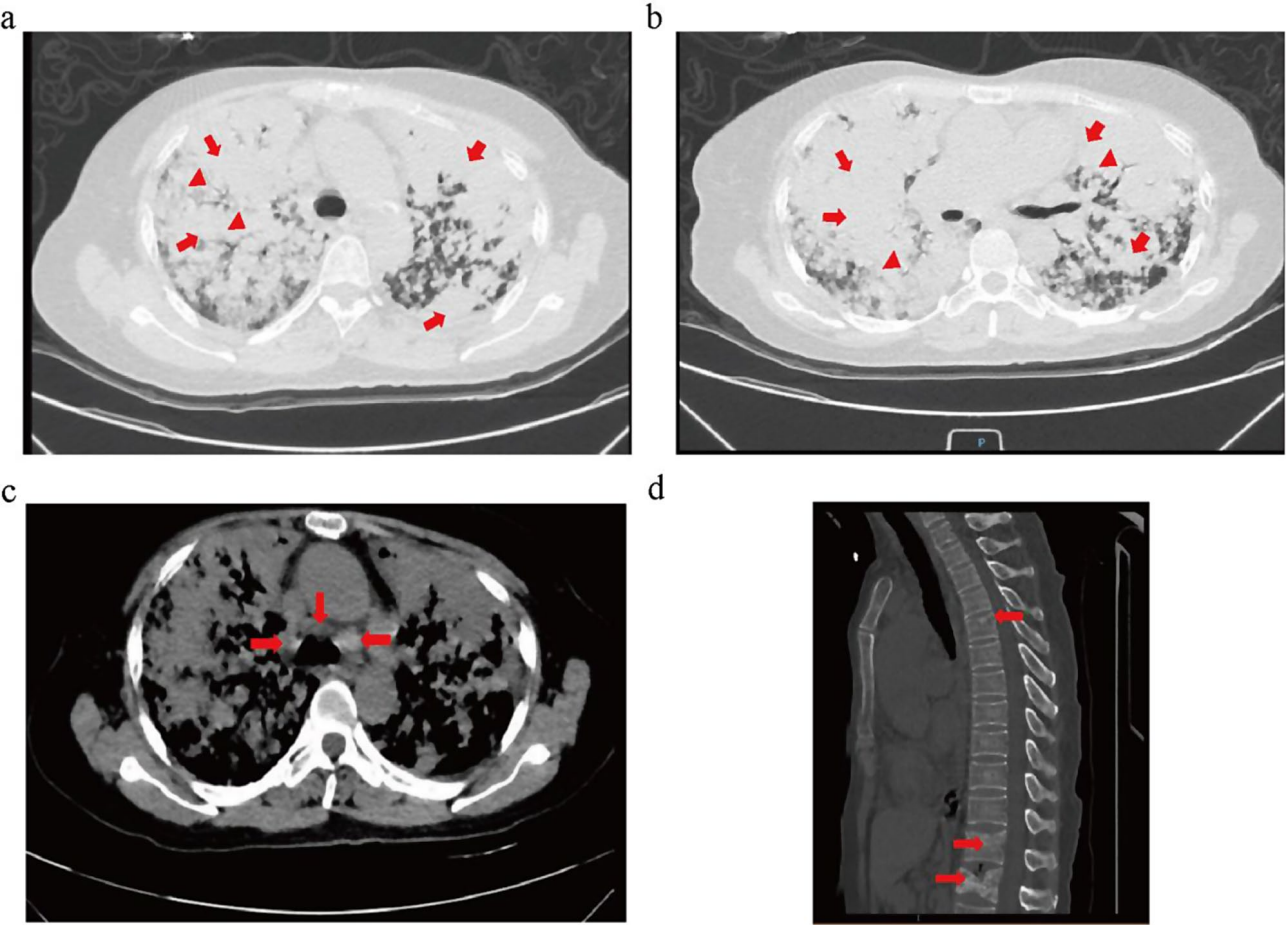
Chest computed tomography (CT) images at initial hospitalization. The scan reveals multiple nodules and plaques in both lungs (a, b, red arrow), air bronchial sign (a, b, red triangle), enlarged mediastinal lymph nodes (c, red arrow), and increased density within the thoracic (T5/T12) and lumbar (L1) vertebrae (d, red arrow)
